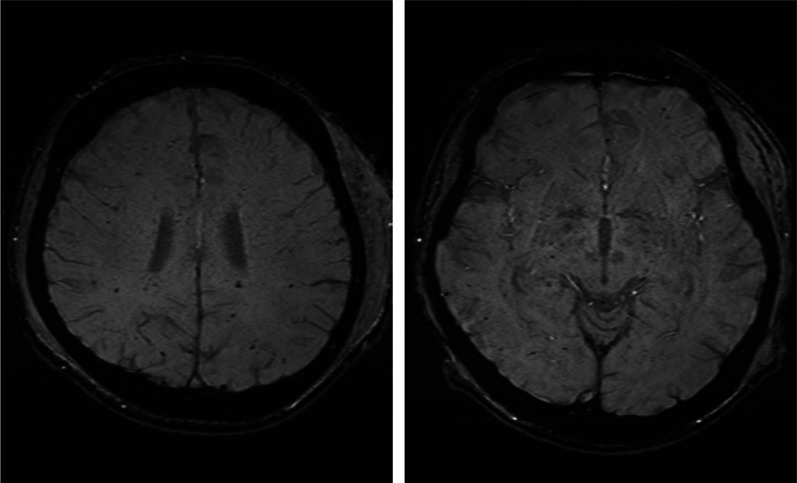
Magnetic resonance images of the brain on the sixth day. Multiple micro-bleeding lesions are observed in the bilateral subcortex, corpus callosum, and bilateral cerebellar hemisphere with susceptibility-weighted images
